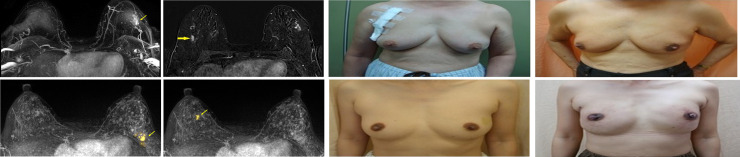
(a-h) Case A: A 75-year-old patient was mammography diagnosed with left breast tumor primarily but the contralateral lesion was found via MR Imaging. She received a bilateral endoscopy assisted partial mastectomy. The final pathology proved the infiltrating ductal carcinoma (IDC) in the right. (e-h) Case B: A 49-year-old patient with pathologic diagnosed of ductal carcinoma in situ (DCIS) of left breast. The contralateral lesion was found via MRI before operation. According to her family history of breast cancer, she received an endoscopic assisted bilateral nipple-sparing mastectomy with immediate breast reconstruction. The final pathology proved bilateral DICS.
